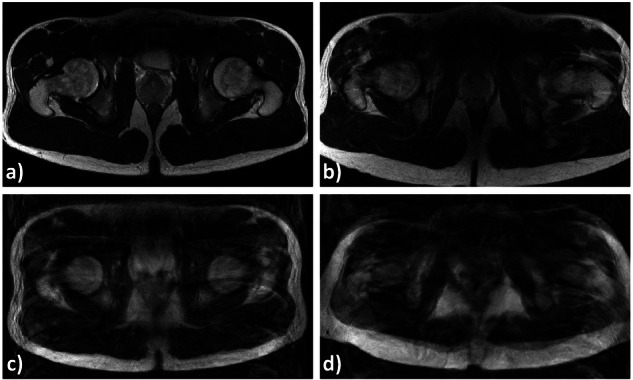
Representative MR images showing motion artifact-induced image degradation. Axial T2-weighted non-fat-saturated images of the pelvis, displaying both hips in different participants, are shown. The images were evaluated for motion artifact-induced degradation and categorized as showing no (a), mild (b), moderate (c), and severe (d) degradation
